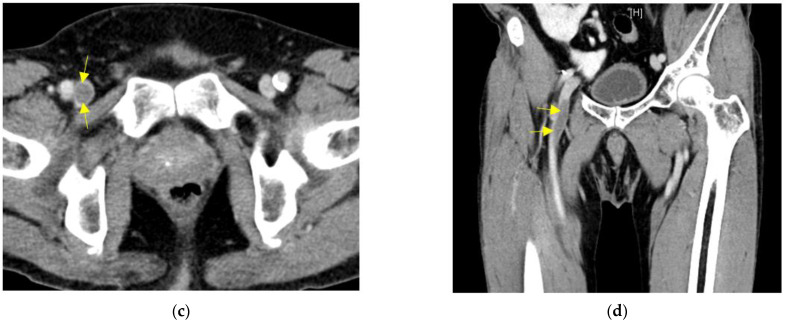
CT of chest and extremity: (a,b) left pulmonary artery thrombosis; (c,d) right femoral vein thrombosis. Arrow indicates thrombosis.
